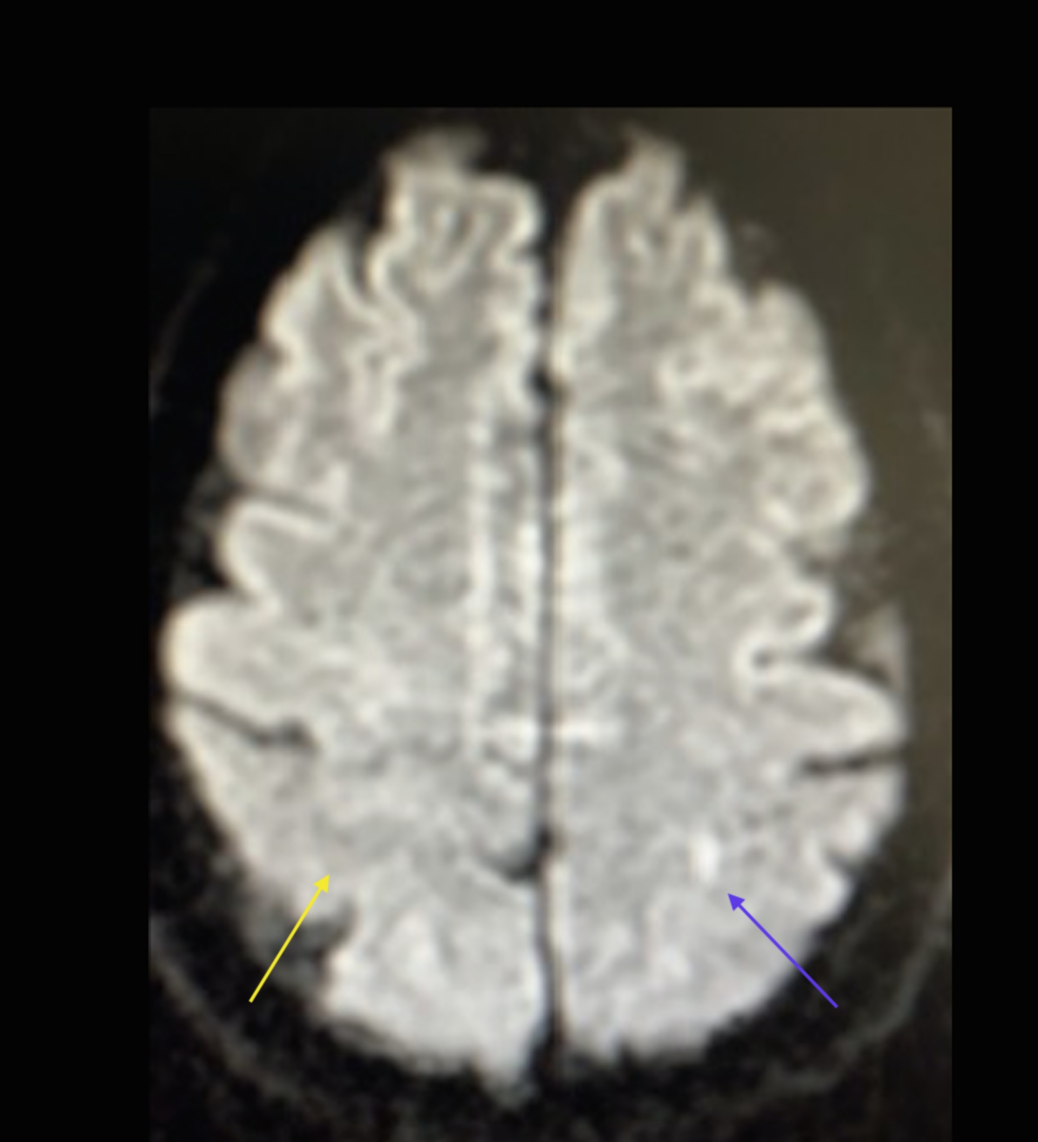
Diffusion-weighted sequence: 0.3 tesla MRI The image shows a faint outline of right hemisphere omega sulcus (yellow arrow) and left-hand knob gyrus ischemic infarct with obscuring of omega sulcus (blue arrow); the resolution was limited by the strength of the magnet MRI: magnetic resonance imaging
